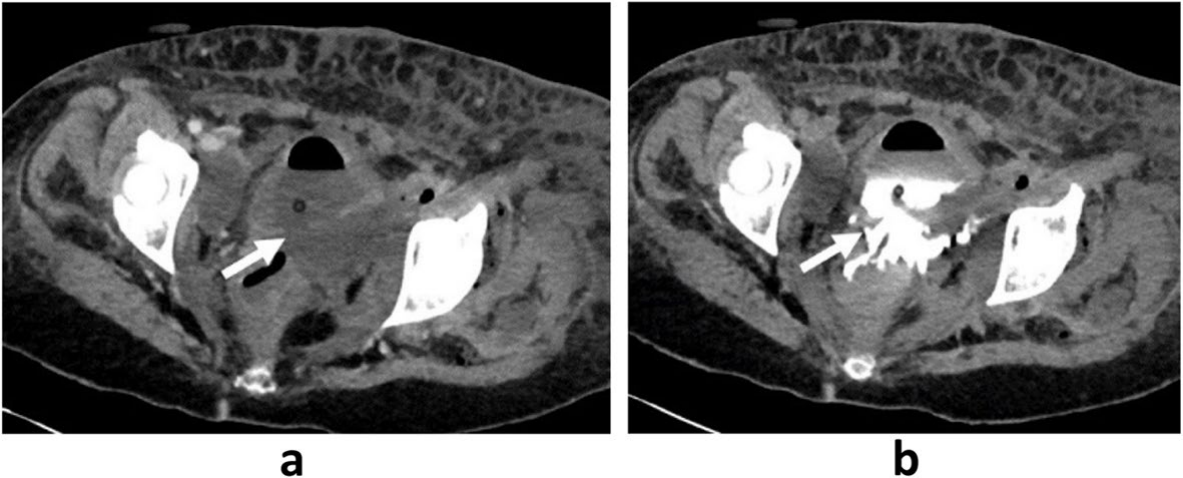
Axial section of a CT scan injected at the venous (a) and excretory (b) phase: rupture of the bladder wall (arrow)
